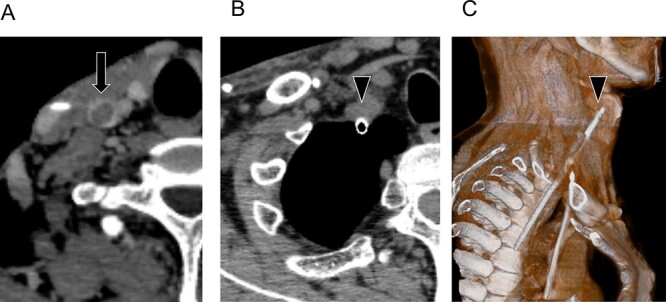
(A) Enhanced CT shows the thrombus in the IJV (arrow). (B) Intrathoracic perforation of the IJV by the introducer (arrowhead). (C) Three-dimensional CT shows the intrathoracic perforation of the introducer (arrowhead).
